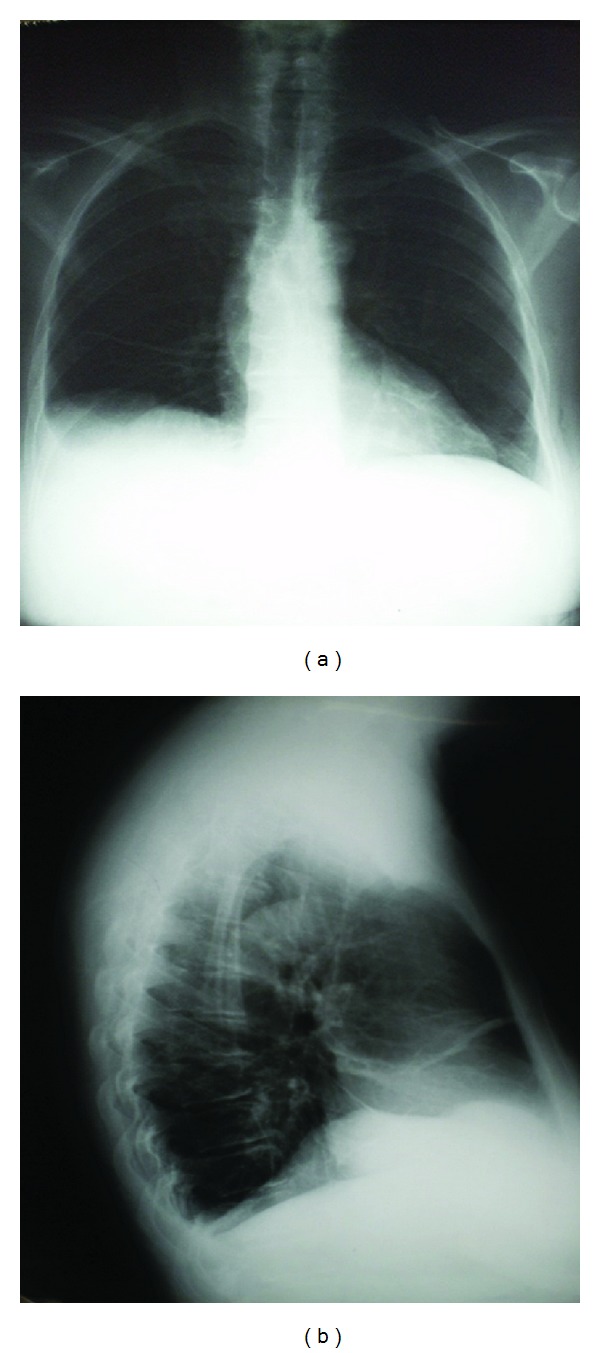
A 57-year-old woman with diffuse large B-cell lymphoma and peritoneal lymphomatosis chest radiography shows right-sided pleural effusion without mediastinal enlargement or other significant abnormalities.
